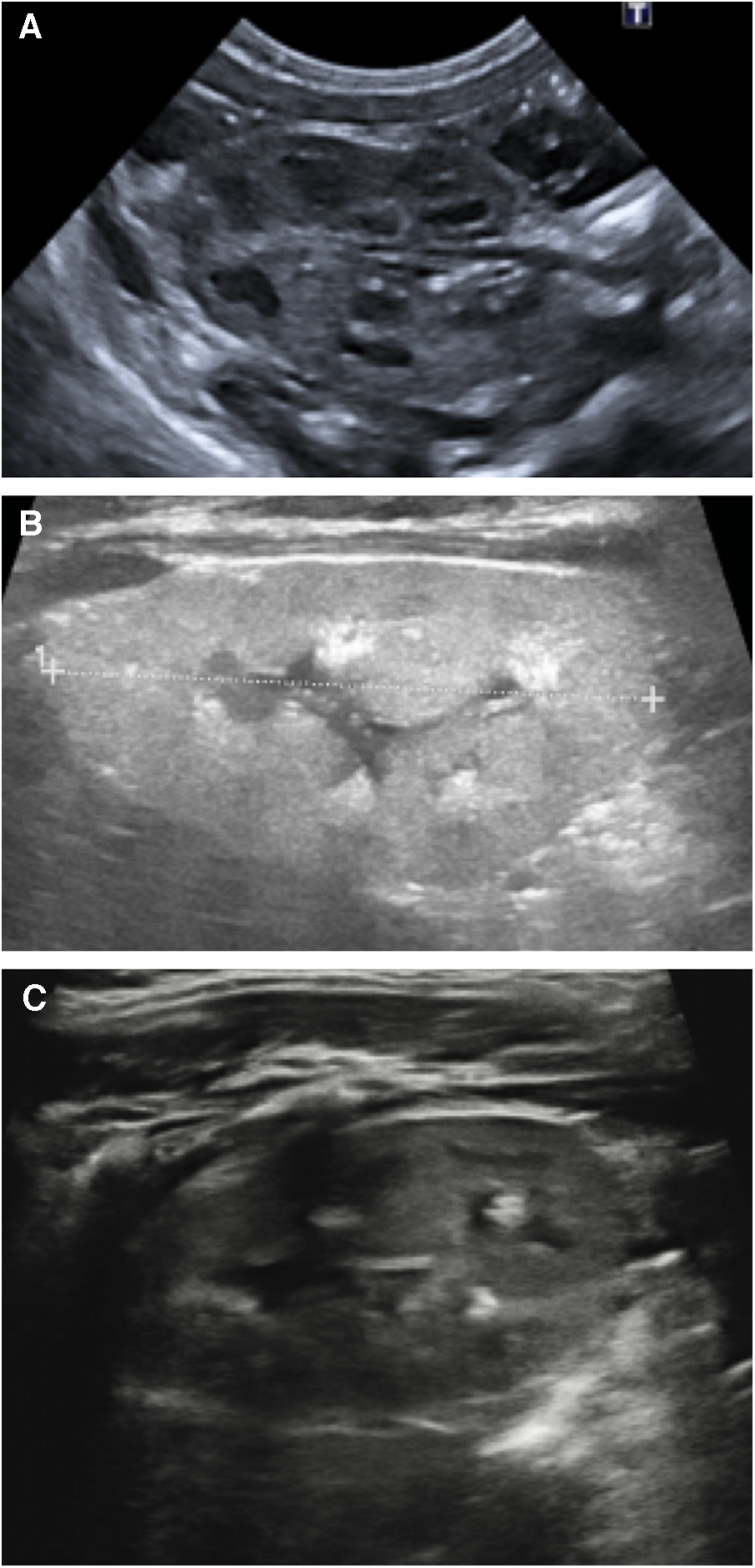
(A) Ultrasound scan at birth; (B) ultrasound scan at 3 months of age; and (C) ultrasound scan at 9 months of age.
